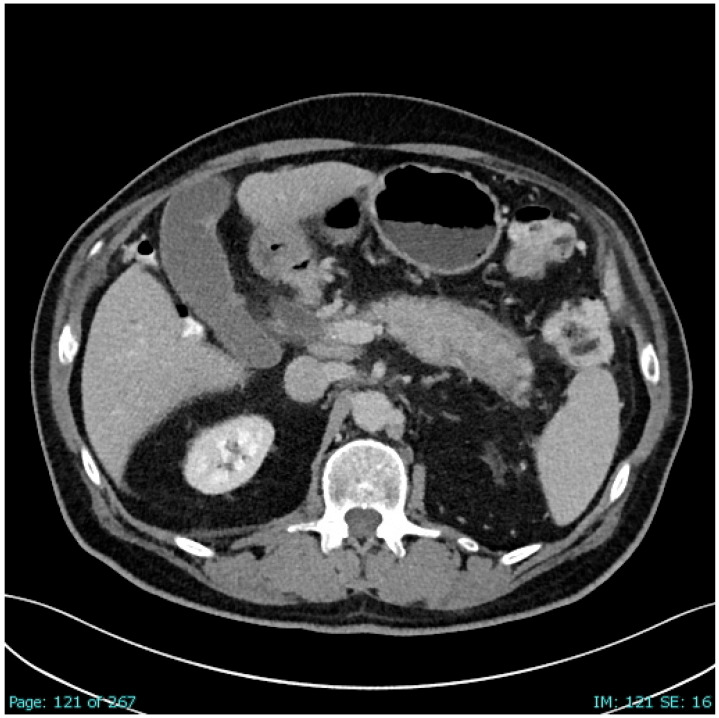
First CT scan of the patient with AIP-4. The computed tomography (CT) scan of the AIP-4 patient was obtained from a previous report published in an open-access journal [2]. The scan shows the portal-venous phase of the enlarged pancreatic tail with sausage-like appearance and a transverse halo sign in the middle right-sided of the scan, representing a scan that is typical and specific for autoimmune pancreatitis.
